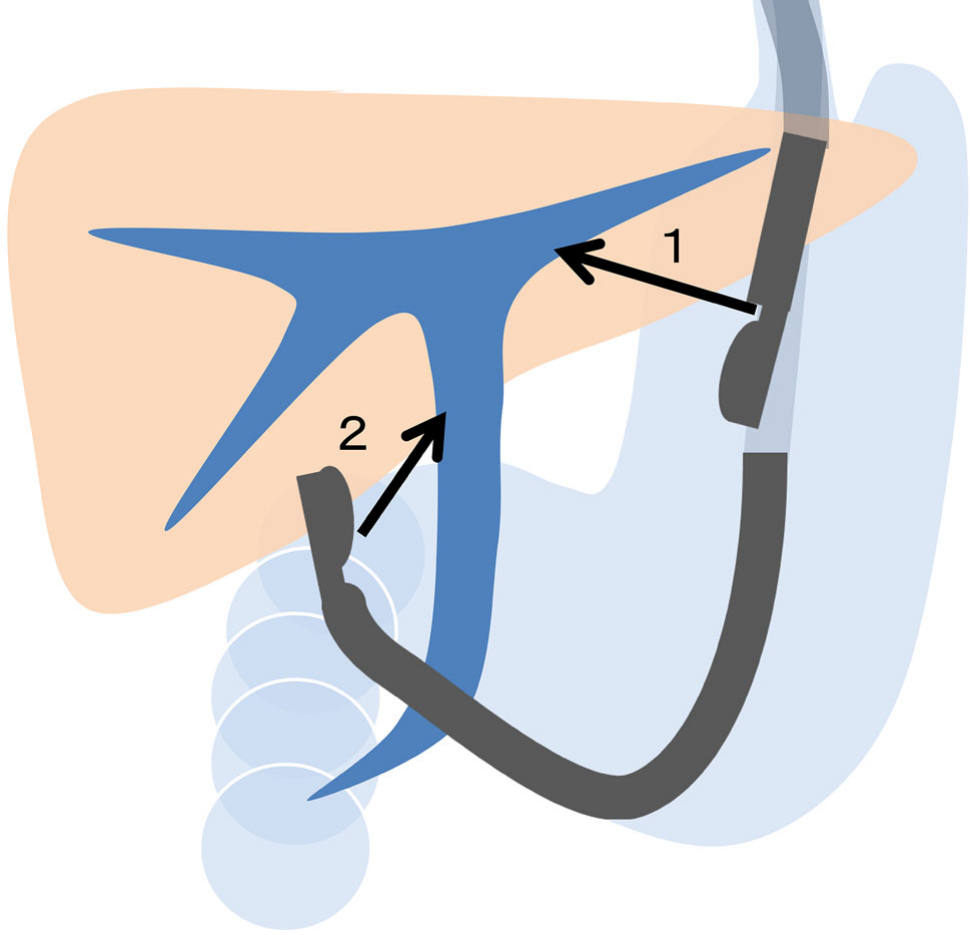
Access routes of endoscopic ultrasound-guided transluminal biliary drainage. *1* Hepaticogastrostomy; *2* choledocoduodenostomy
